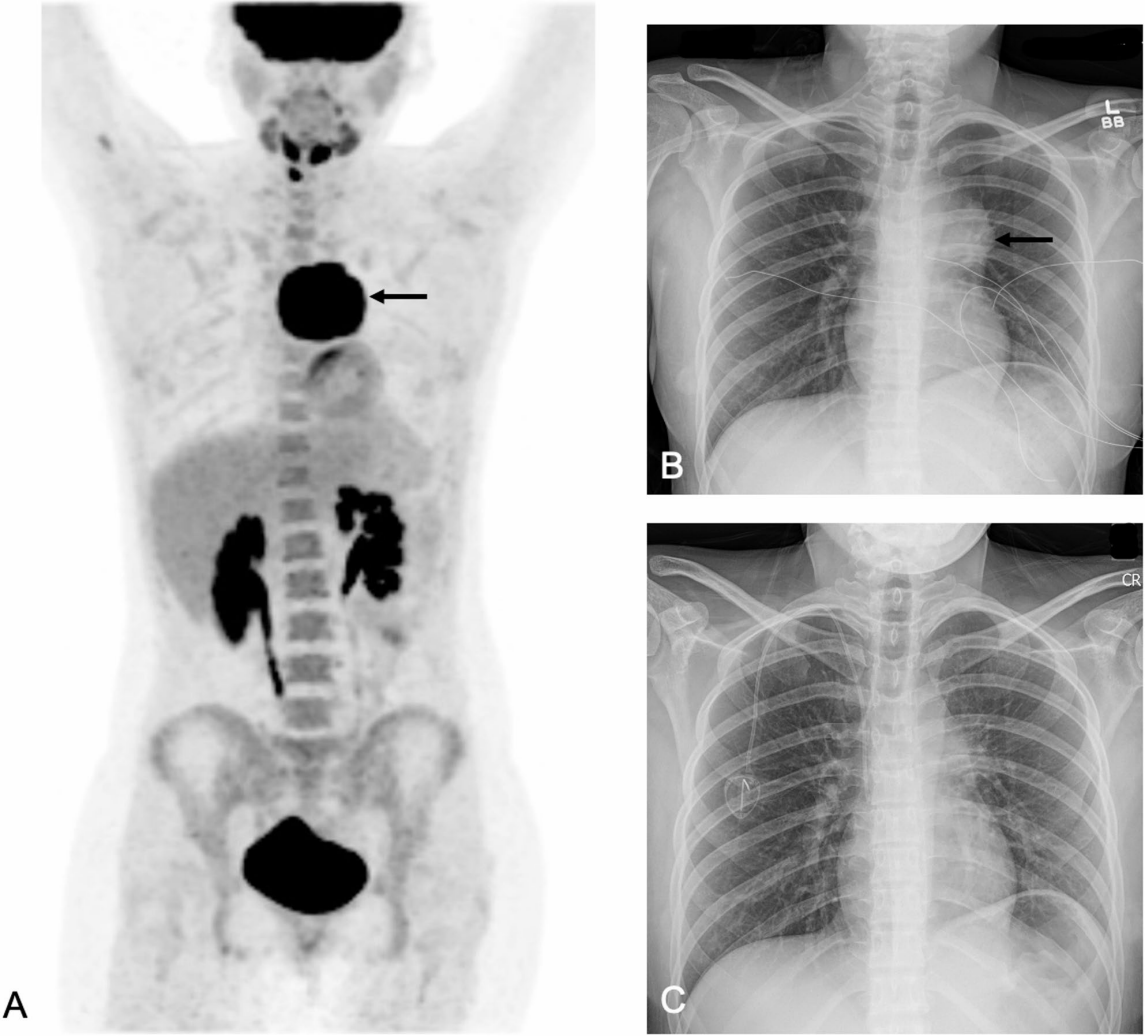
19-year-old female with mediastinal large B cell lymphoma and relatively low metabolic tumor burden at baseline who did not develop CRS or ICANS after CAR T-cell therapy. A) Baseline PET MIP shows anterior mediastinal mass (black arrow). No other lesions were noted. B) Baseline Chest radiograph demonstrates mild widening of the mediastinum, consistent with the mediastinal mass (black arrow). C) Chest radiograph on day 9 after the CAR T-cell injection demonstrates mild widening of the mediastinum. No new pleural effusion or pulmonary infiltrates, consistent with an uncomplicated post–CAR T-cell clinical course
